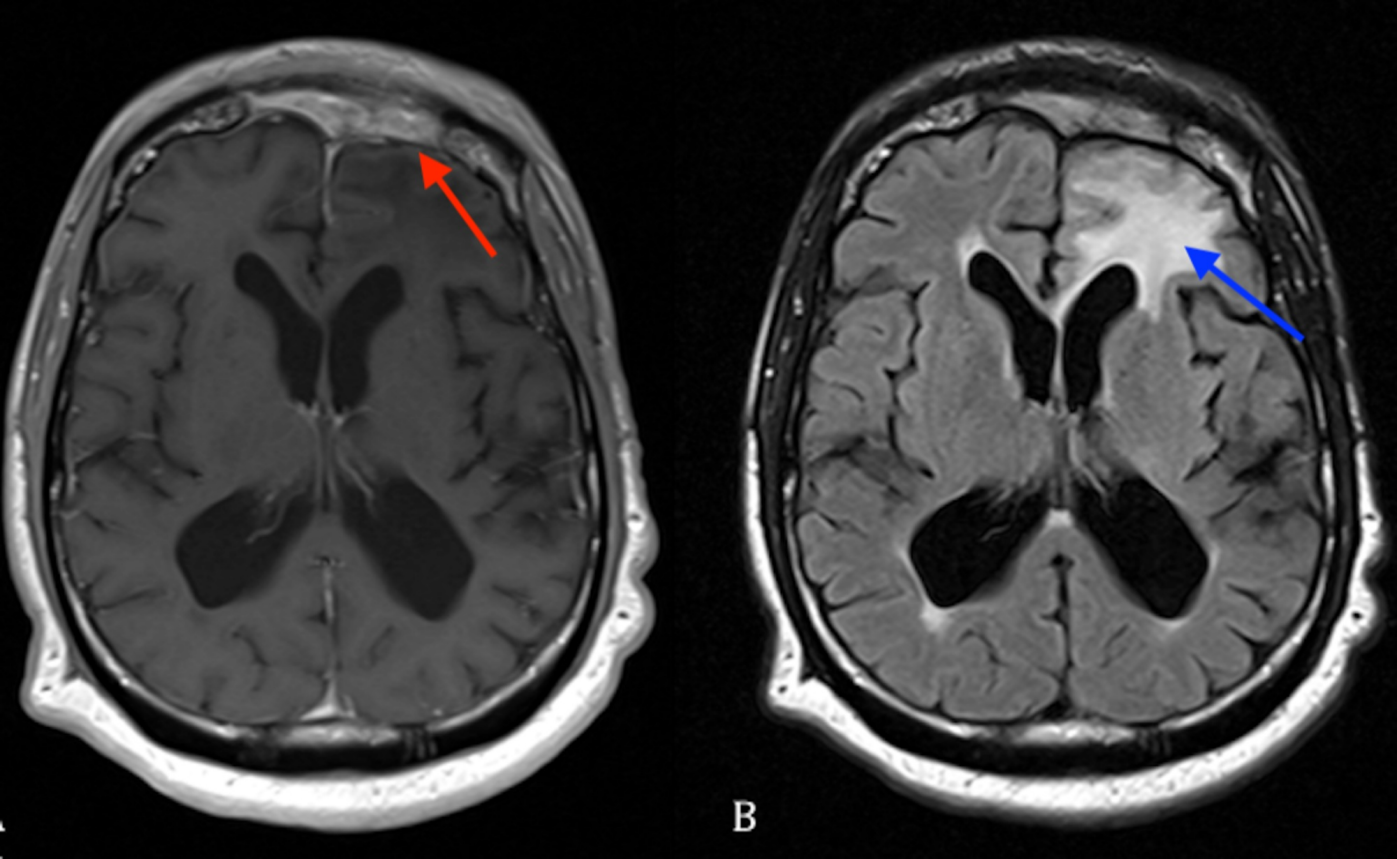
(A) Axial T1 MRI with contrast showing resolution of infection (red arrow). (B) Axial FLAIR MRI showing resolution of infection with some residual flair changes (blue arrow). FLAIR: Fluid-attenuated inversion recovery; MRI: Magnetic resonance imaging.
